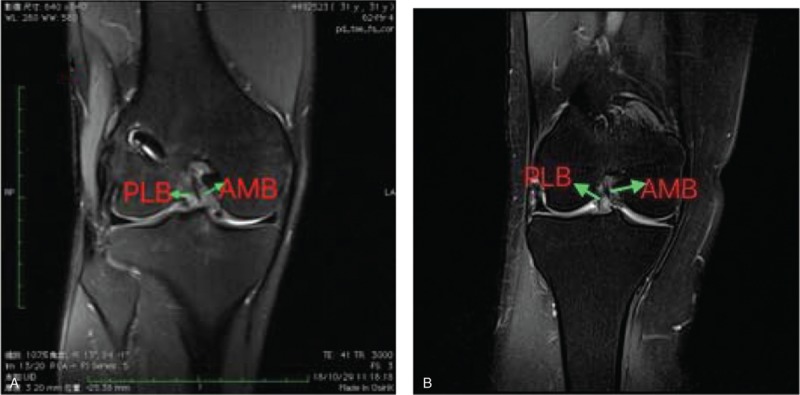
On oblique coronal MRI, the 2 bundles were seen clearly. a:postoperation; b: healthy people. MRI = magnetic resonance imaging.
